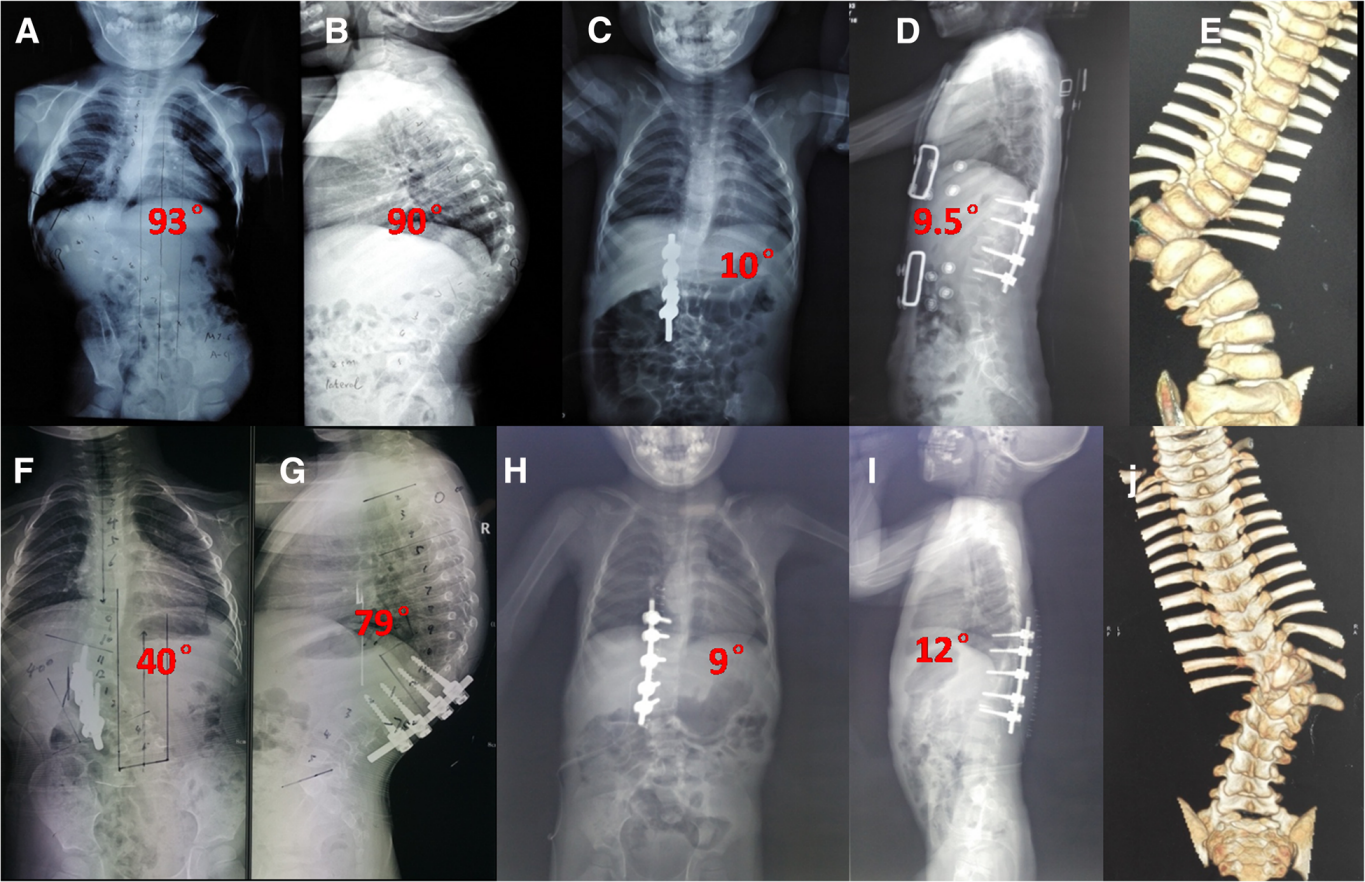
A 2.5-year-old boy with T12 hemivertebra. Radiograph images and 3D-computed tomography images obtained preoperatively (a, b, e, j), postoperatively (c, d), and at the latest follow-up visit, 12 months later (f, g) and revision surgery (h, i)
